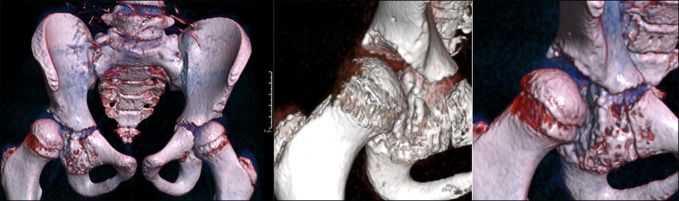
Three-dimensional reconstructed CT imaging showing intra-articular acetabular lesion
